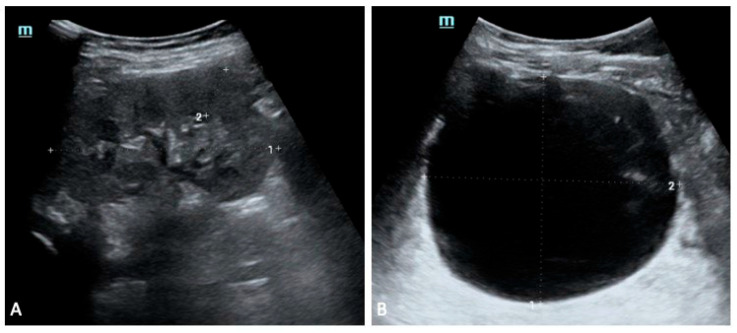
Sibling 2. Abdominal ultrasound exam performed at 5 years of age; images exploring the right (A) and left (B) perirenal spaces of the abdominal cavity. (A) shows a normal-sized kidney [pole-to-pole diameter approximately 79 mm (dotted line 1), anterior parenchymal thickness of 17 mm (dotted line 2)], with normal echogenicity. On the other hand, the left kidney structure is diffusely altered with several cysts seen within, ranging in size from a few millimeters to some centimeters (the most prominent one approximately measures 81 mm × 91 mm in the sagittal plane (dotted lines 1 and 2 in (B)).
